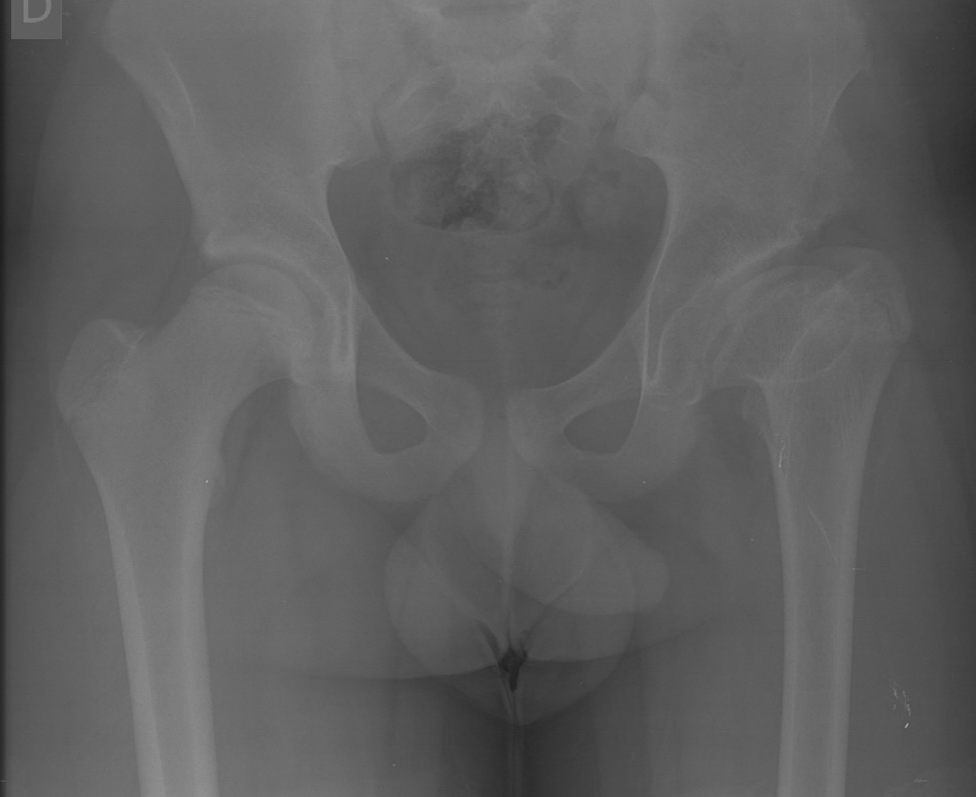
Preoperative radiograph showing morphological sequelae of Legg-Calvé-Perthes disease in the left hip.
